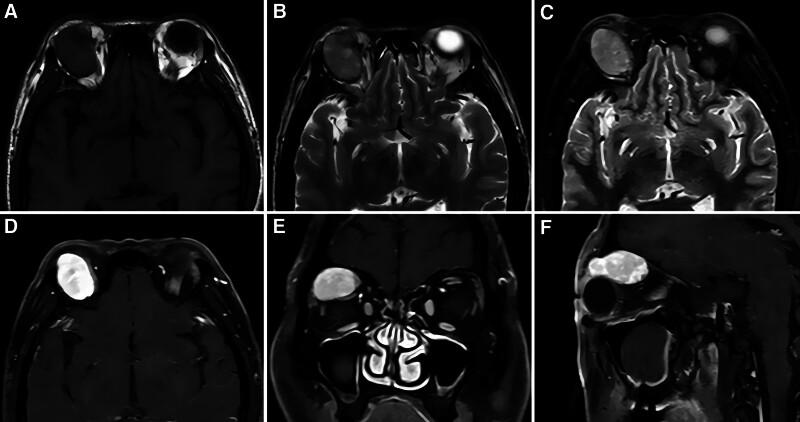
CE-MRI images. T1WI, the tumor was hypointense (A); T2WI, the tumor was slightly hyperintense (B); T2WI–SPAIR showed high signal (C); CE-MRI showed significant uneven enhancement of the tumor (D–F). CE-MRI = contrast-enhanced magnetic resonance imaging.
